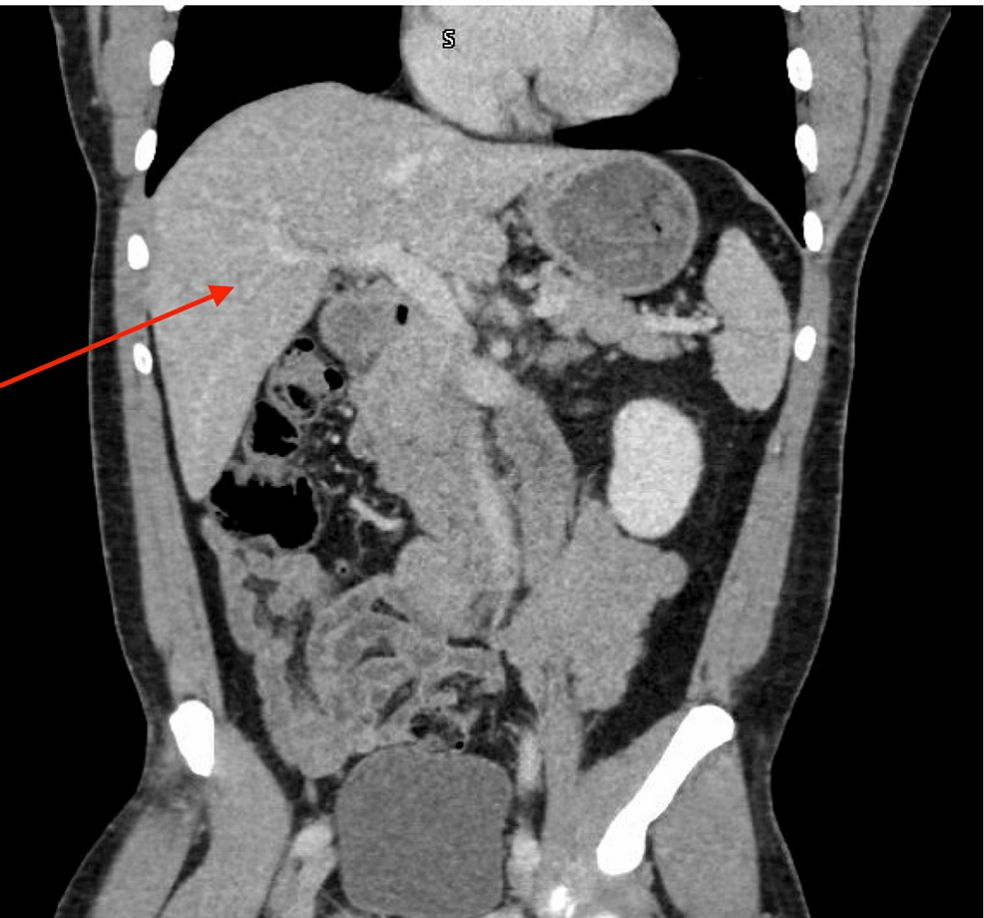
Computed tomography (CT) abdomen/pelvis with contrast showing no hepatobiliary pathology; red arrow shows a normal-sized liver without any masses, nodules, or fibrotic changes.
